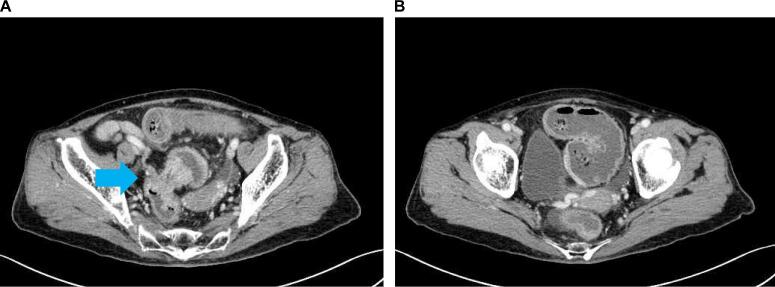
Computed tomography findings. In the rectosigmoid junction, thickening of the wall with contrast enhancement (arrow) and dilatation of the colon proximal to the sigmoid colon were observed.
